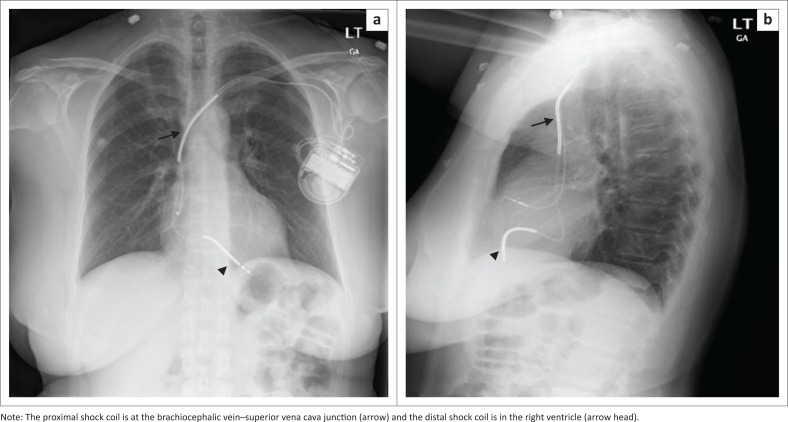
Chest radiographs posterior–anterior (a) and lateral views (b) showing a dual-lead implantable cardioverter defibrillator with its characteristic shock coils implanted in a 62-year-old female patient with non-ischaemic cardiomyopathy.
